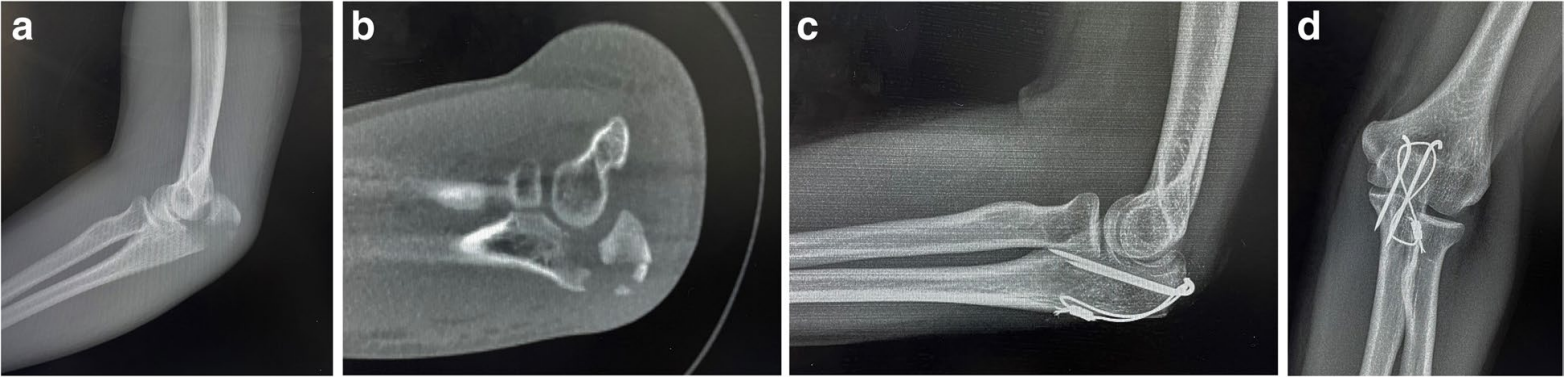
A 36-year-old male underwent surgery with TBW. a X-ray film of the lateral position of the elbow joint before the operation; b Plain CT scan of the elbow joint before the operation; c d Postoperative anteroposterior and lateral X-ray film of the elbow joint
